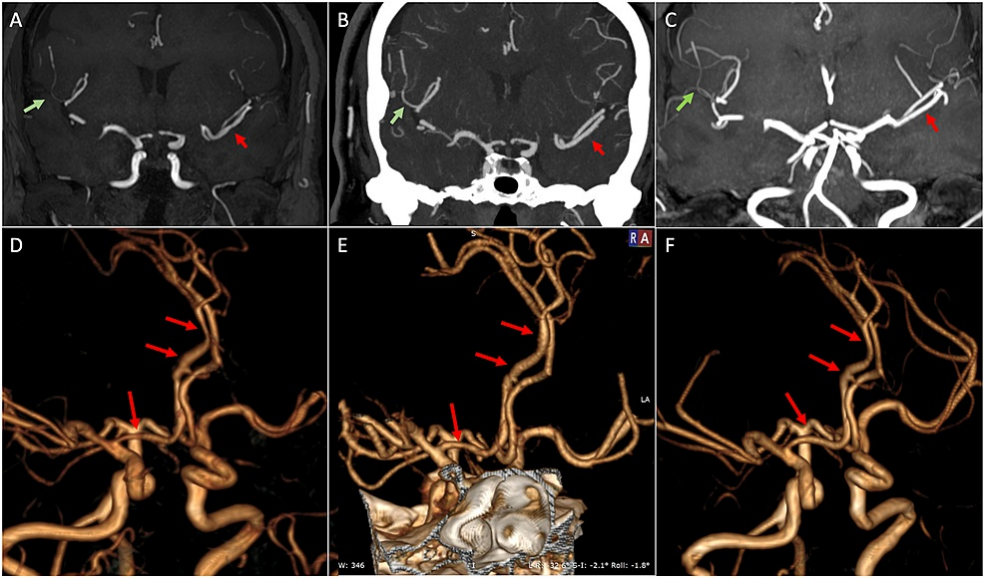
Segmental stenosis of intracranial branches (A, D): TOF angiography performed at 15 days of illness shows irregularity due to segmental stenosis of the ventral branch of the left MCA (red arrow) and of a right M4 cortical artery (green arrow). Irregularities with areas of stenosis and dilations of the right anterior cerebral artery are also observed. (B, E): In the CT angiography performed 21 days after the onset of the disease, there is complete resolution of the stenosis in the ventral branch of the left MCA and partial resolution in the right cortical artery. In the TOF angiography performed at three months (C, F), there were no findings of vasoconstriction.
